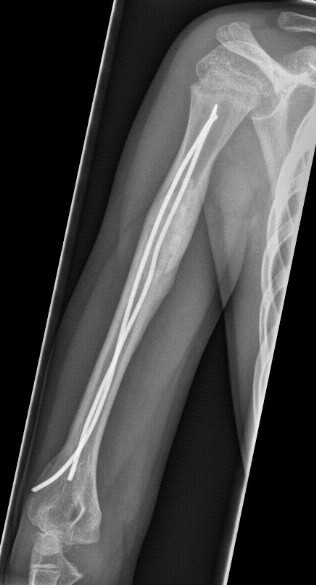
Patient presenting with acute pathologic fracture. Consolidation with ESIN, Orthoss^® ^and GPS^®^. Lateral view X-ray five months after treatment with ESIN, Orthoss^® ^and GPS^®^. As a consequence, Nails could be removed.
